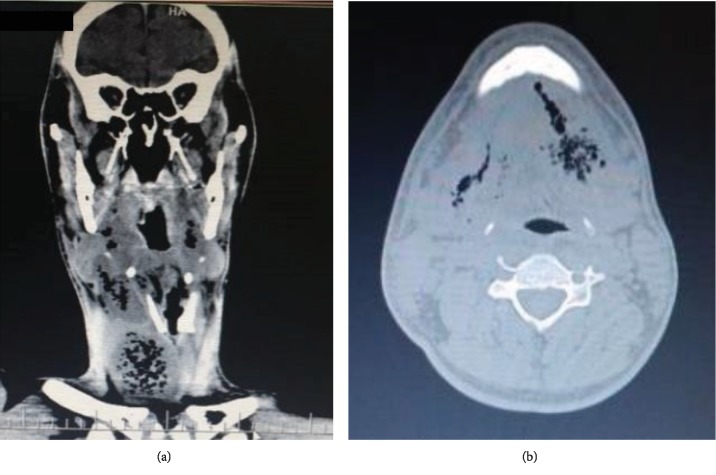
(a, b) Computed tomography of the face and cervical region revealing soft tissue enlargement associated with subcutaneous emphysema between muscular planes. Purulent mediastinal collections with gaseous contents and tracheal deviation can be seen.
